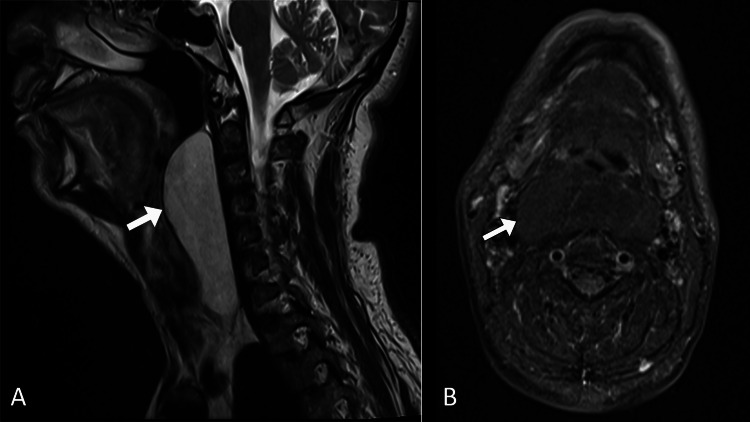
MR images of the neck in the sagittal (A) and axial (B) planes showing a well-defined retropharyngeal lesion (arrows) with a high signal intensity on T2-weighted image (A) and complete suppression on fat-suppressed sequence (B). MR: magnetic resonance.
